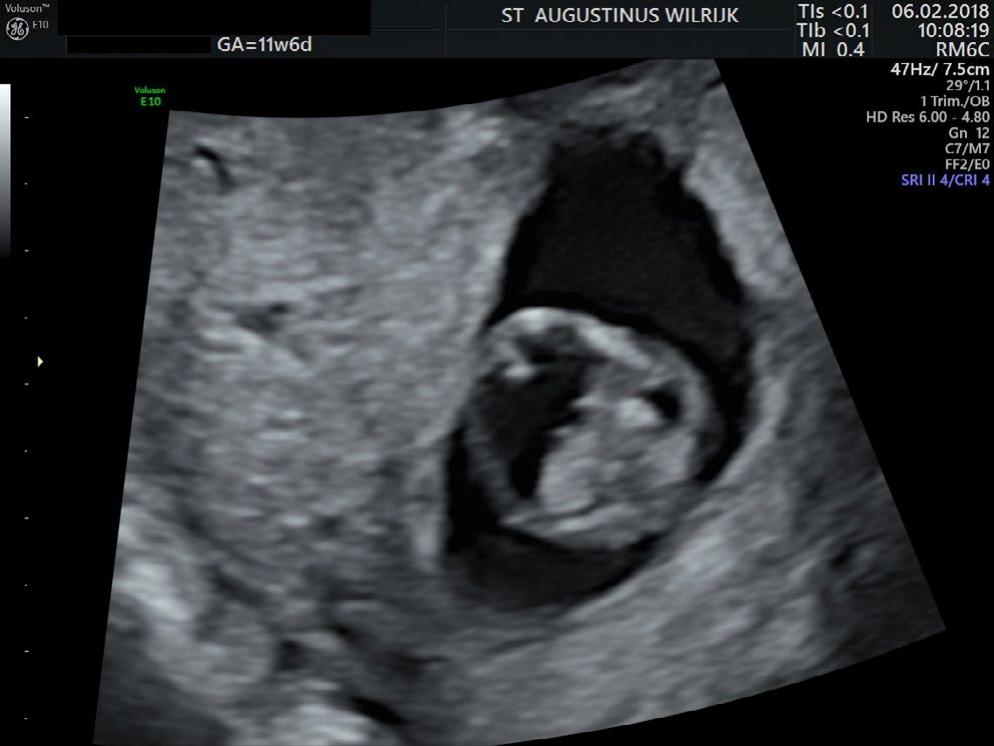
Ultrasound at 11 wks and 6 d shows an abnormal cerebral anatomy, with preferential diagnosis of holoprosencephaly
